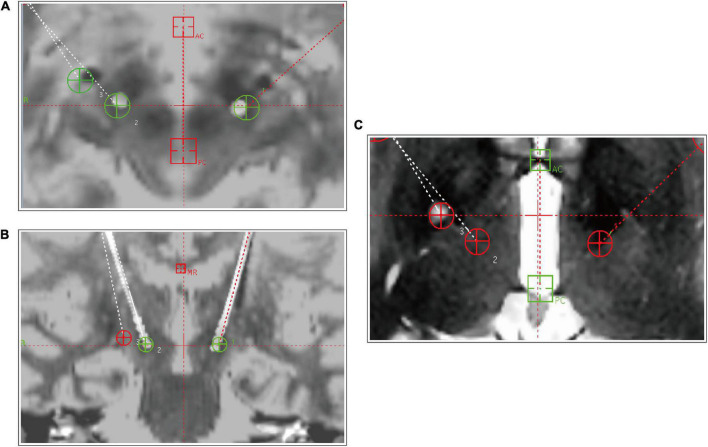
Postoperative MR images of patient 5, demonstrating positions of the implanted electrodes in the bilateral STN (A,B) and unilateral pallidotomy. The two red orthogonal lines refer to the Cartesian coordinate system in each view, whereas the diagonal lines, with or without green circles, represent the trajectories of the implanted leads. In the center of each view, the two green circles (named 1 and 2) in (A,B) show the planned targets, and the one red circle (named 3) in (C) shows the position of pallidotomy. AC, anterior commissure; MR, midline reference; PC, posterior commissure.
